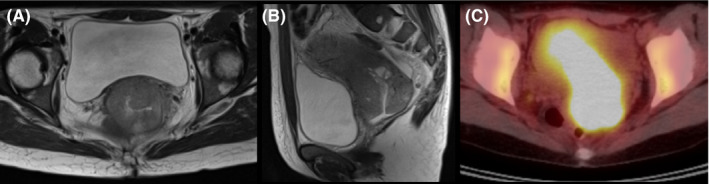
Magnetic resonance imaging (MRI) and ^18^fluoro‐2‐deoxyglucose‐positron emission tomography (FDG‐PET) showing the primary tumor before the initiation of chemoradiotherapy. A, MRI axial plane. B, MRI sagittal plane. C, FDG‐PET axial plane
